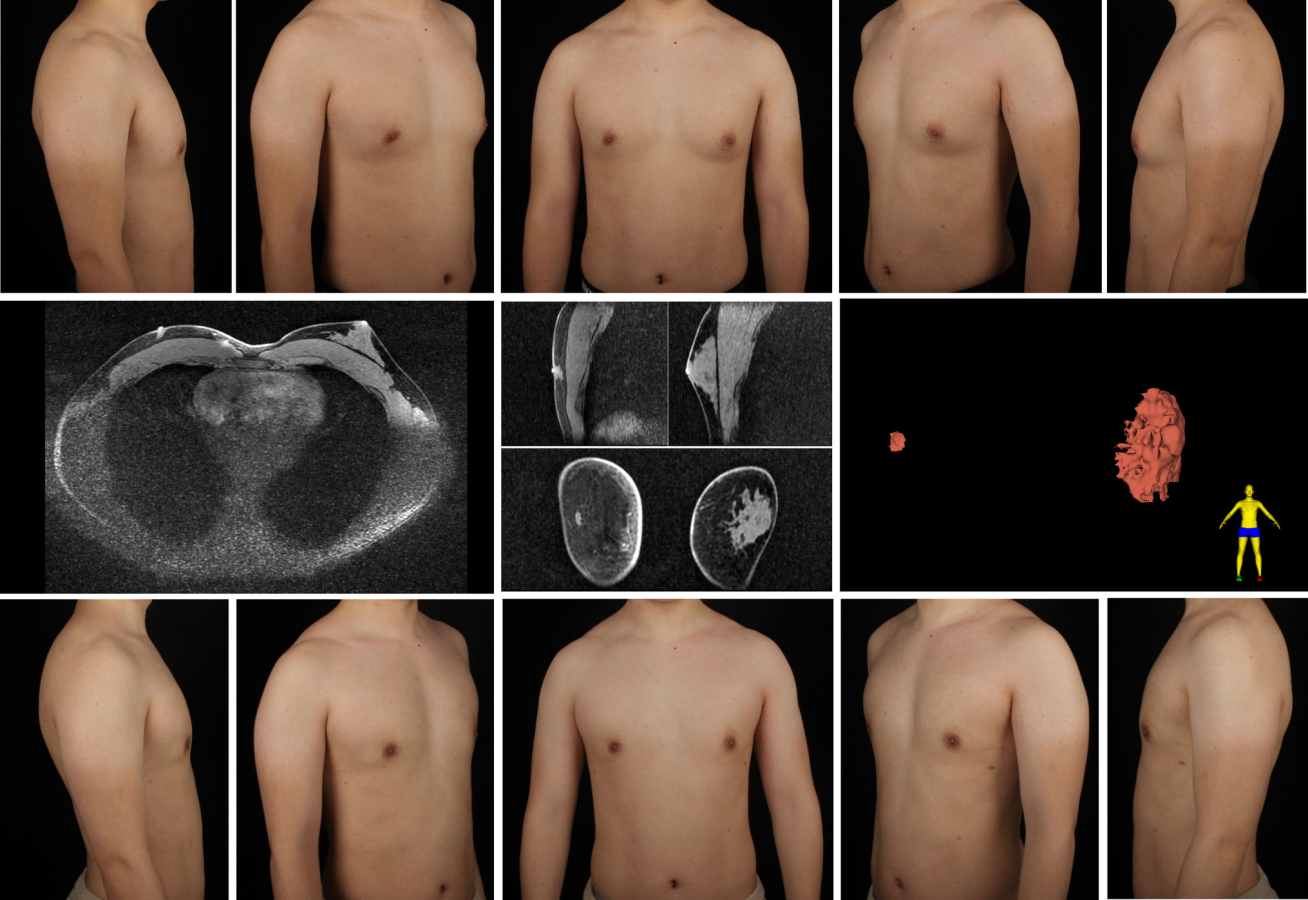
Asymmetric gynecomastia. A 29-year-old man showed grade 1/type 1C (right side) and grade 2/type 2C (left side) gynecomastia, based on chest appearance (upper) and MR images (middle). Liposuction alone was performed on region 1 of right chest, while both mammotome-assisted resection and liposuction were performed on region 1–2 of left chest. Chest appearance 6 months after surgery (lower)
